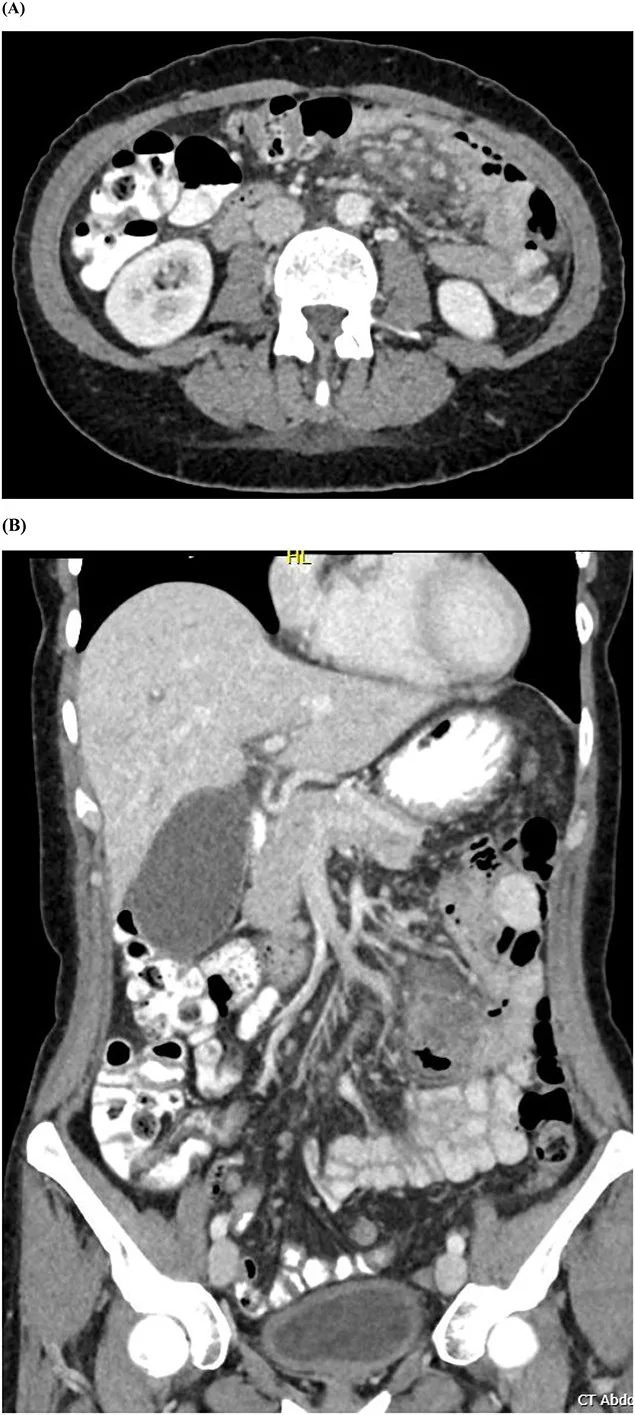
Oral and intravenous contrast-enhanced CT of the abdomen on Day 4. (A) Axial and (B) coronal images demonstrate a focally abnormal segment of jejunum in the left mid-abdomen with marked mural thickening, submucosal oedema, and surrounding mesenteric inflammatory change. No extraluminal contrast, free gas, or drainable collection was present. The findings were considered atypical and the preoperative differential diagnosis included jejunal diverticulitis and devascularized small bowel.
